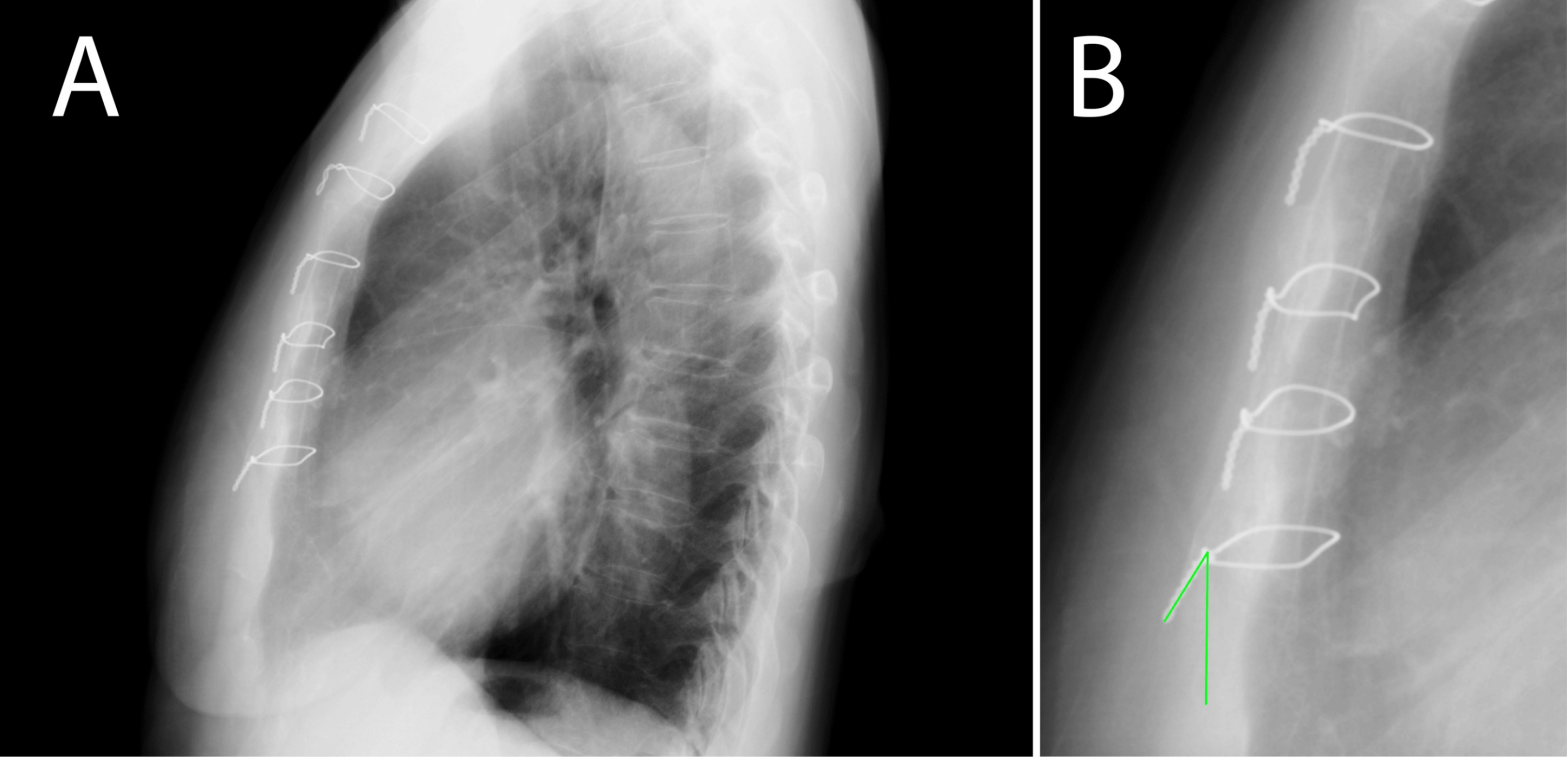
Chest X-p after the first operation (A) Lateral chest X-p reveals the sixth wire standing up. (B) The angle between the vertical line is 31.4° (green line). Chest X-p: chest X-ray photograph
